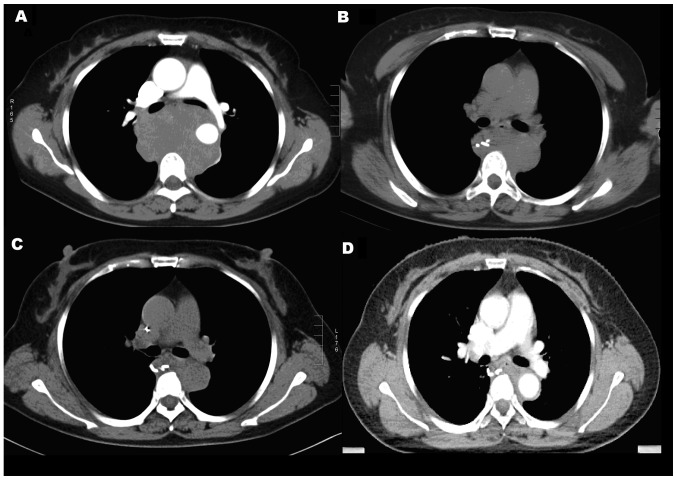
Thoracic computed tomgraphy (CT) scan showing the lesion prior to treatment and during varying stages of treatment. (A) CT scan from December 25, 2012, showing a large mass in the posterior mediastinum. (B) Following one cycle of chemotherapy, the tumor was greatly reduced in size. (C) CT scan showing the smaller tumor following three cycles of chemotherapy. (D) Pre-radiotherapy CT scan showing the residual tumor.
